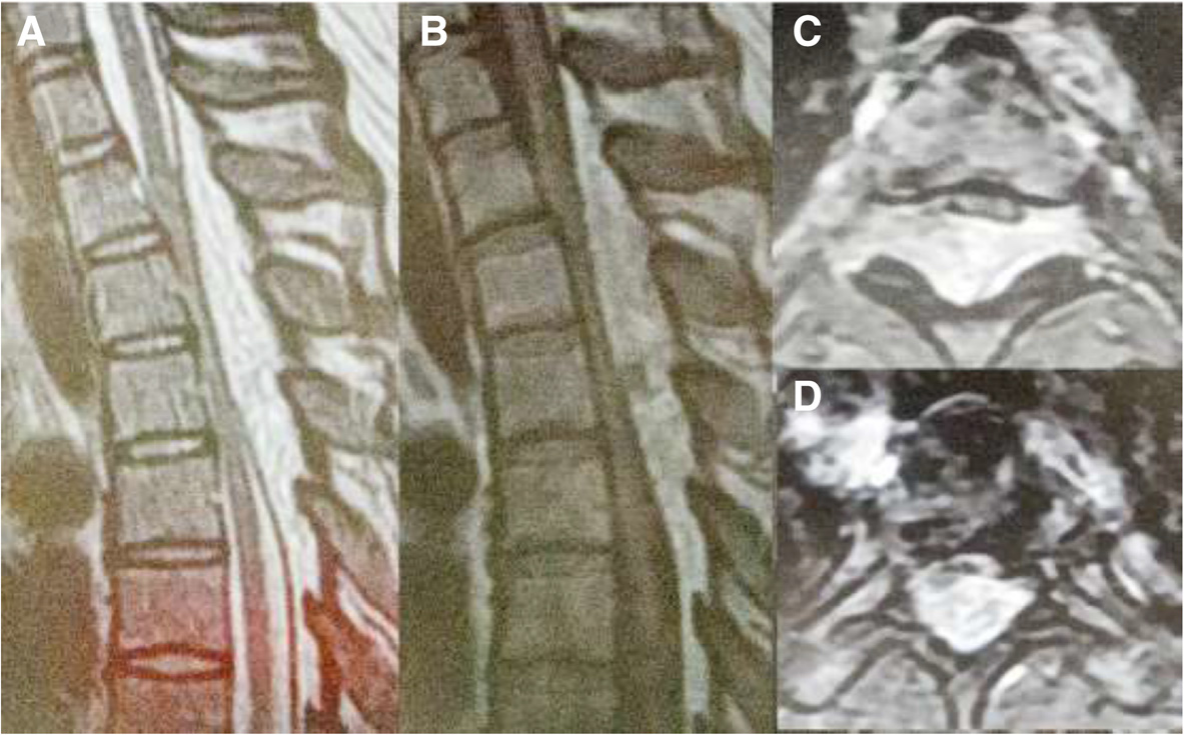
Pre-operative images. (A) Pre-operative sagittal T2-weighted magnetic resonance image and (B) pre-operative sagittal T1-weighted magnetic resonance image showing a mixed epidural mass at the T1 to T6 level. (C) Post-contrast T1-weighted axial magnetic resonance images at the T3 to T4 level and (D) T5 level showing the mass along the posterior epidural spinal canal of the thoracic spine, compressing and displacing the spinal cord anteriorly.
